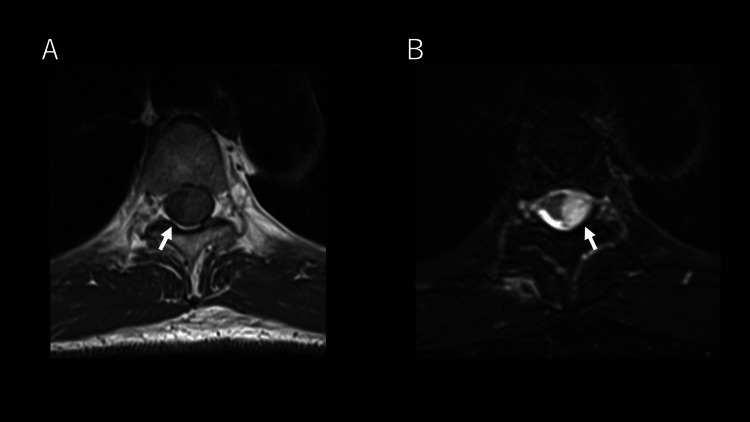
Thoracic spine MRI on the day of admission demonstrated findings suggestive of a spinal subdural hematoma extending from T3/4 to T5/6 (A) T1-weighted image shows no displacement or alteration of the epidural fat, supporting a subdural rather than an epidural lesion. (B) T2-weighted image demonstrates a hyperintense mass-like lesion compressing the spinal cord. Note: The left side of each image corresponds to the patient’s right side. MRI: Magnetic resonance imaging
